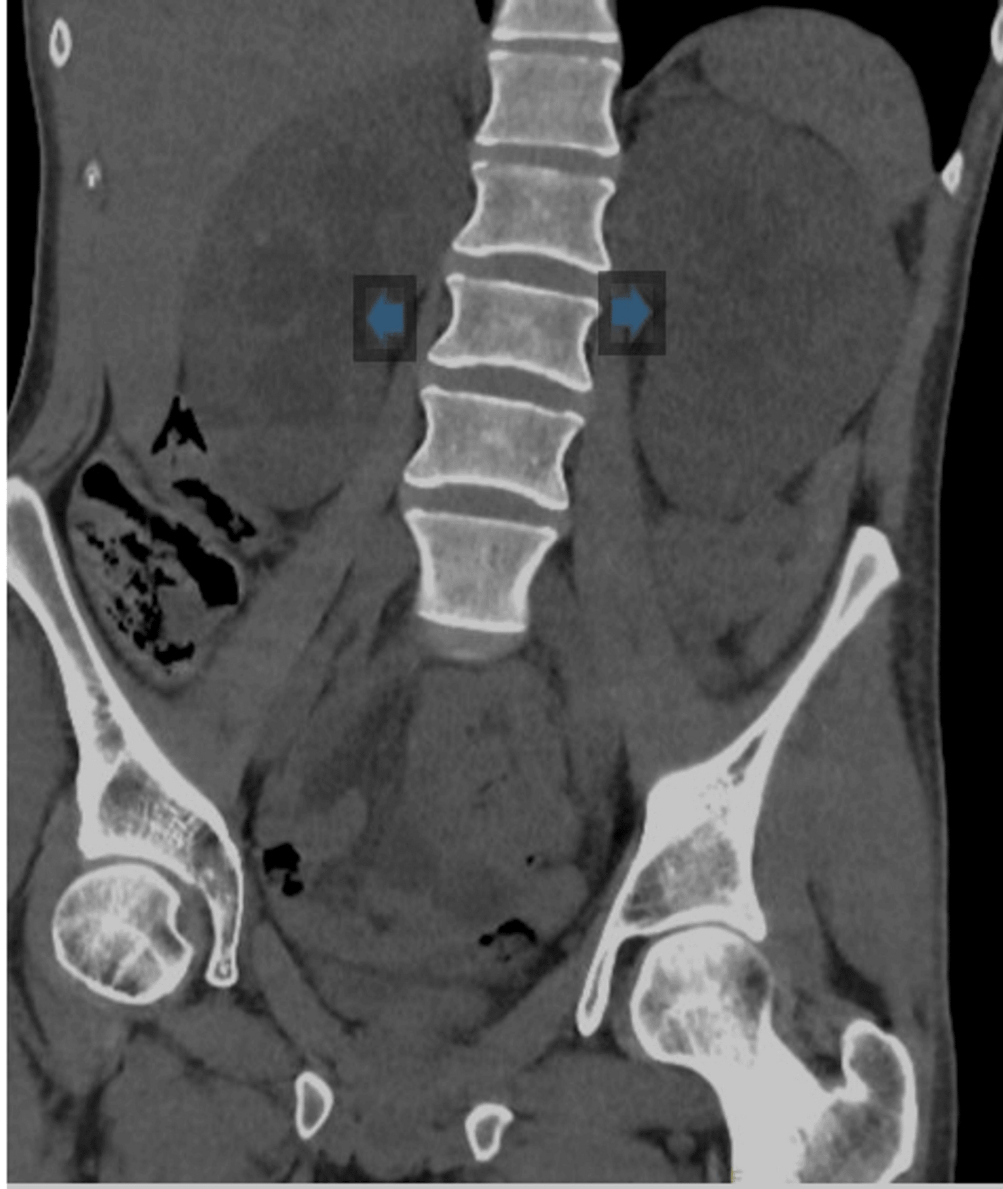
CT renal showing bilateral dilatation of pelvicalyceal system
